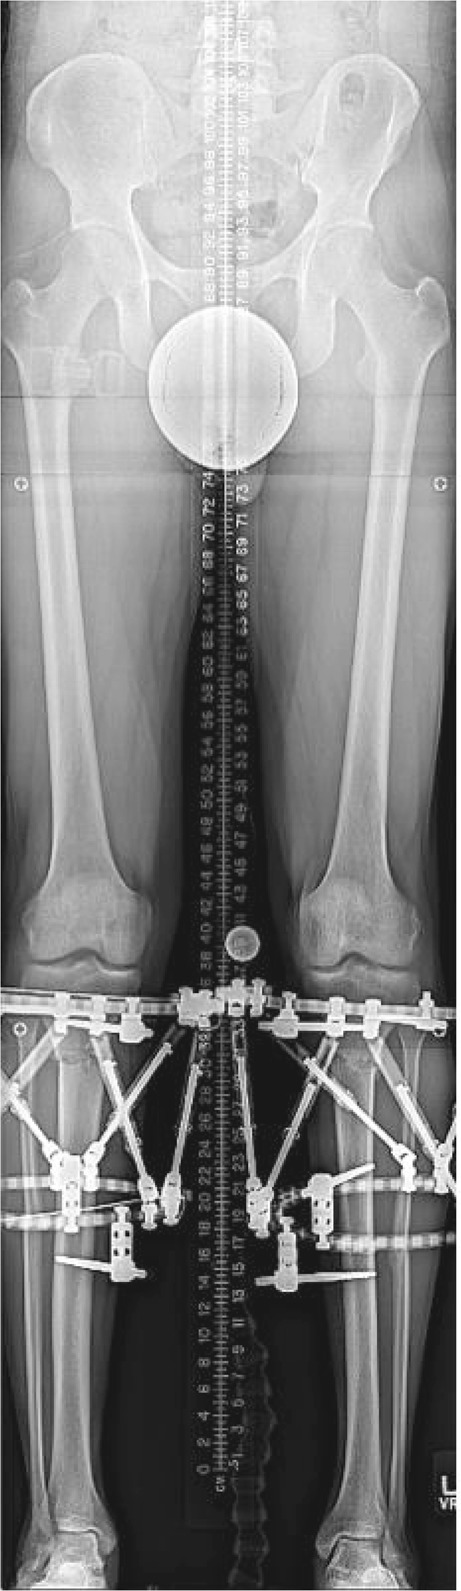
Standing hip-to-ankle radiograph showing neutral alignment once full correction was achieved with bilateral hexapod external fixators in place.
